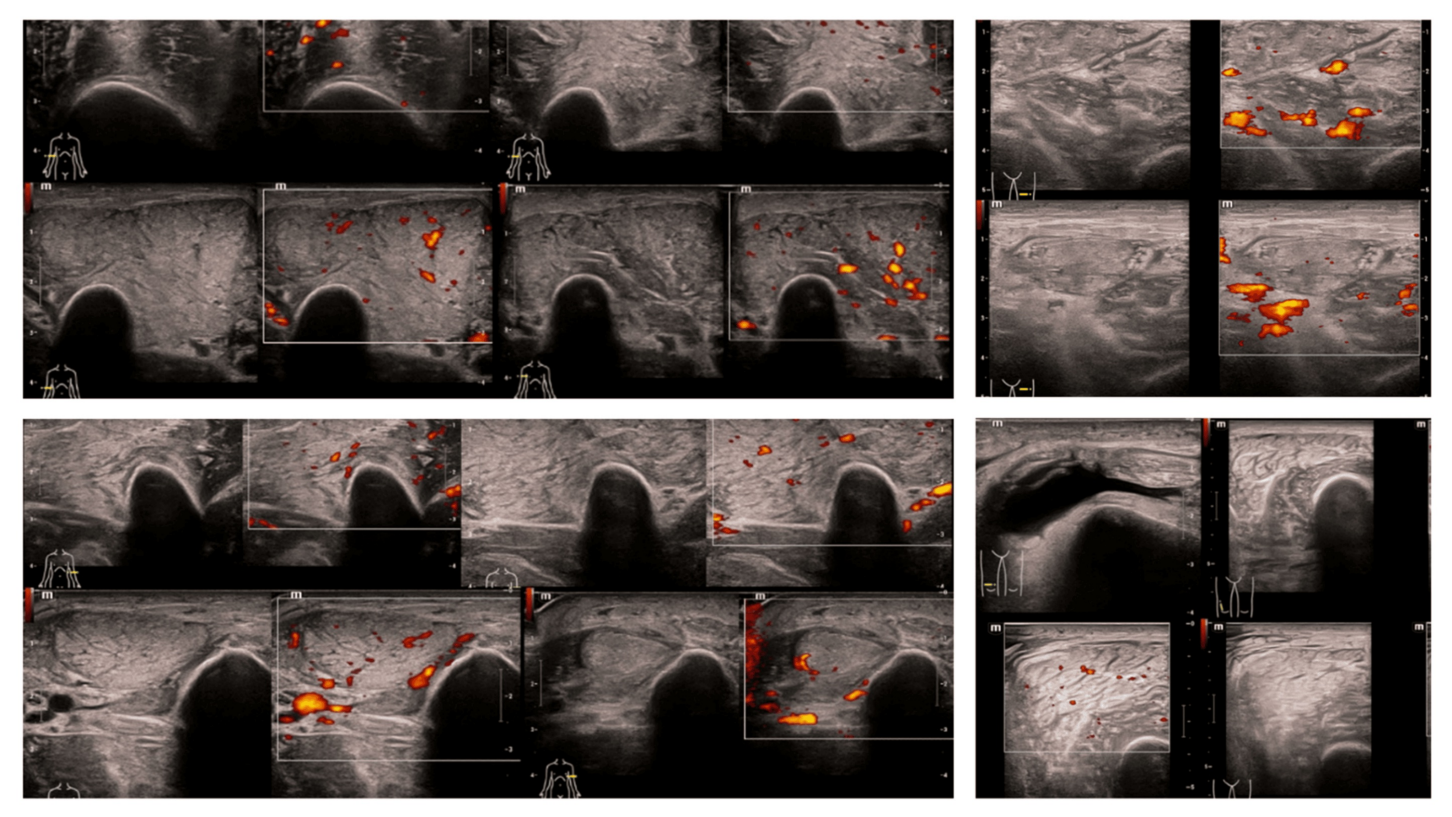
Musculoskeletal ultrasound with color Doppler evaluation of bilateral upper and lower extremity muscle groups A: Transverse view of an upper extremity muscle demonstrating increased muscle thickness and mildly increased echogenicity with scattered intramuscular Doppler signals, indicating active hyperemia; B: Contralateral upper extremity muscle showing a similar pattern of diffuse echogenicity and low-to-moderate intramuscular vascular flow on color Doppler, consistent with symmetric inflammatory involvement; C: Upper extremity muscle with preserved fascial planes but increased intramuscular Doppler activity, suggestive of inflammatory hypervascularity without abscess formation; D: Corresponding contralateral muscle demonstrating patchy vascular signals within thickened muscle fibers, maintaining bilateral symmetry; E: Longitudinal view of upper extremity musculature showing diffuse hypoechoic changes compatible with edema and mild Doppler flow enhancement; F: Similar longitudinal assessment on the opposite side confirming symmetric structural and vascular alterations; G: Lower extremity muscle displaying marked intramuscular Doppler activity with heterogeneous echotexture and increased muscle bulk; H: Contralateral lower extremity muscle with moderate-to-marked hypervascularity and preserved deep fascial contours; I: Lower extremity muscle demonstrating multifocal Doppler signals within edematous muscle fibers, without evidence of fluid collection; J: Longitudinal lower extremity view showing architectural distortion secondary to inflammatory edema, without venous thrombosis; K: Prominent Doppler signal within lower extremity musculature, reflecting active inflammatory hyperemia; L: Bilateral comparison image demonstrating diffuse increased echogenicity and mild vascular activity, consistent with systemic inflammatory myopathy
